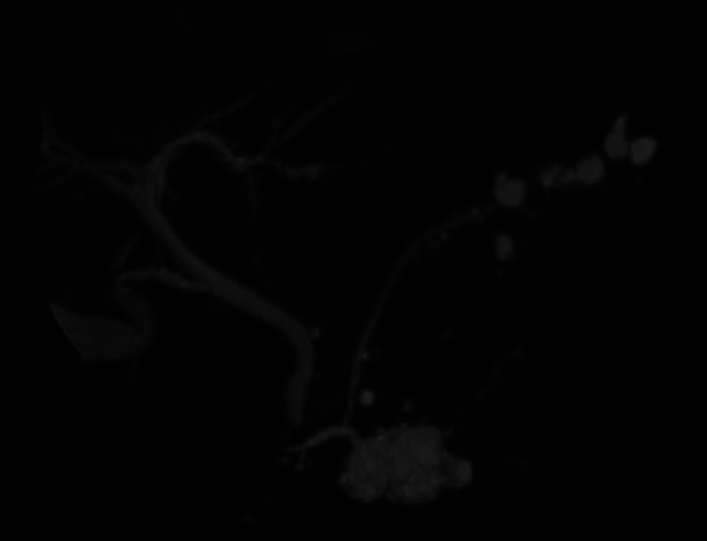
Magnetic resonance imaging (MRI) with magnetic resonance cholangiopancreatography of the abdomen demonstrating lobulated cysts in the pancreatic uncinate process with few thin septations and communication with the main pancreatic duct, suggestive of side-branch intraductal papillary mucinous neoplasms (SB-IPMN)
